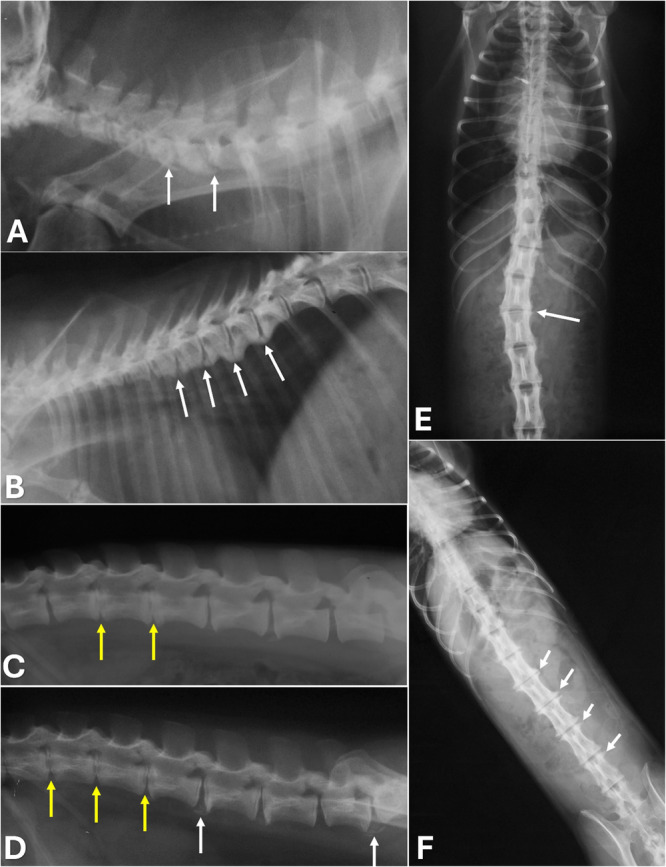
Radiographic images of the vertebral column in the *Chlorocebus aethiops*. (A) Cervical segment, lateral view: ventral spondylosis with ankylosis tendency (arrows). (B) Thoracic segment: ankylosing ventral spondyloses (arrows). (C) Lumbar spine, lateral view: reduced intervertebral spaces (yellow arrows) and mild spondylosis (white arrows). (D) Lateral view: endplate sclerosis and narrowed intervertebral spaces (yellow arrows). (E) Ventrodorsal view: thoracolumbar scoliosis (arrow). (F) Ventrodorsal view: lateral spondylosis in multiple lumbar vertebrae (arrows).
